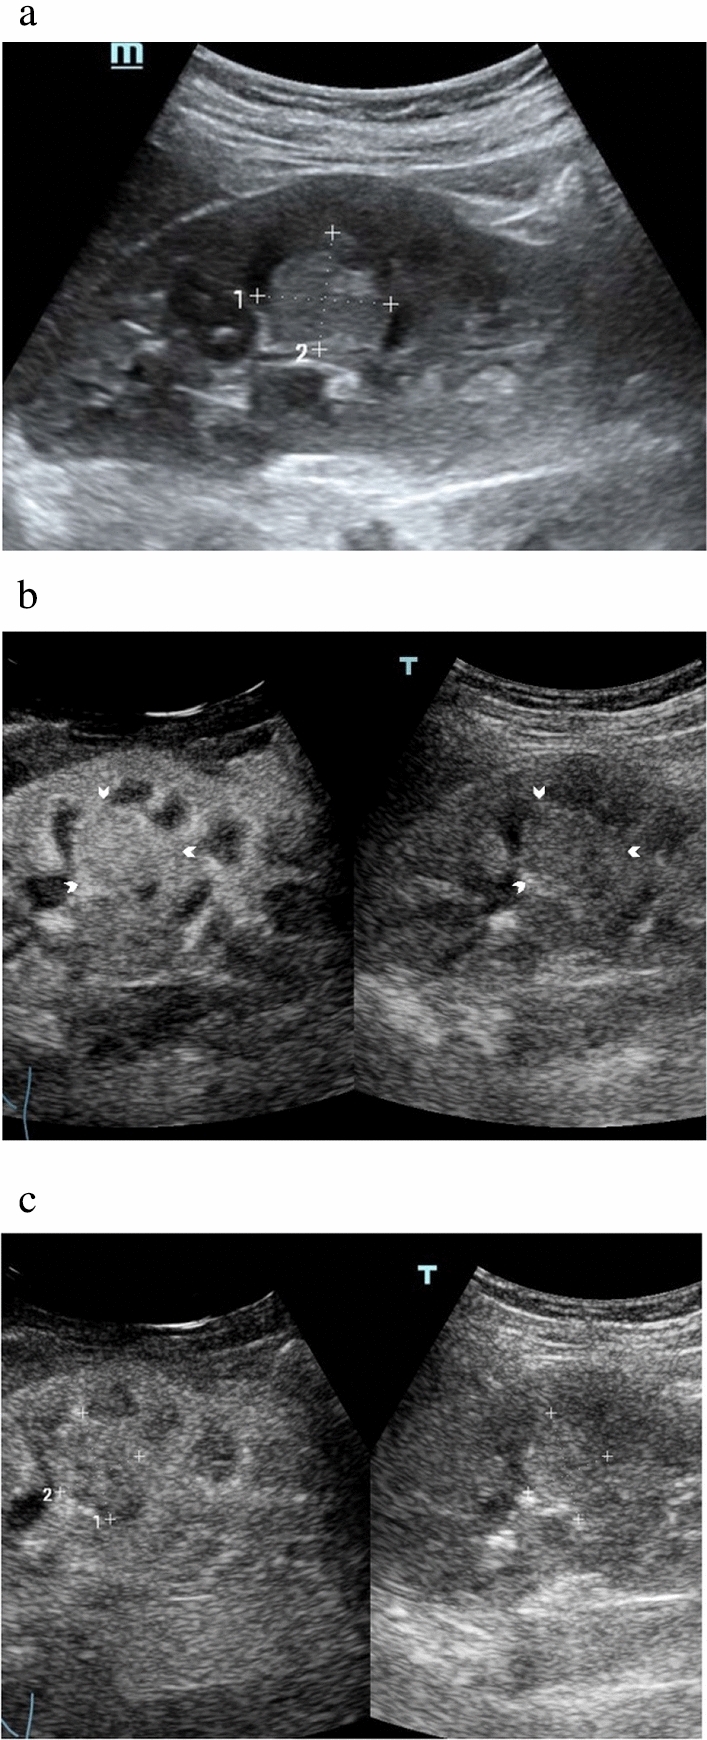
Images in a 17-year-old girl with confirmed clear cell renal cell carcinoma. (a) Gray-scale ultrasound shows a solitary 2.4-cm homogeneous hyperechoic lesion (calibers) in the right kidney. Contrast-enhanced ultrasound images of the lesion demonstrate (b) homogeneous slightly hyperenhancement in cortical phase (17 s) (arrowheads) and (c) isoenhancement in parenchymal phase (130 s) (calibers).
